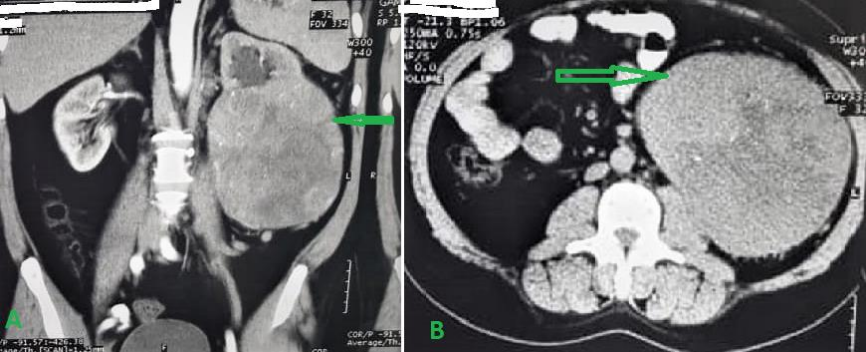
abdominal computed tomography scan showing the left renal mass with solid and cystic composition in axial (A) and coronal (B) views (arrows)
